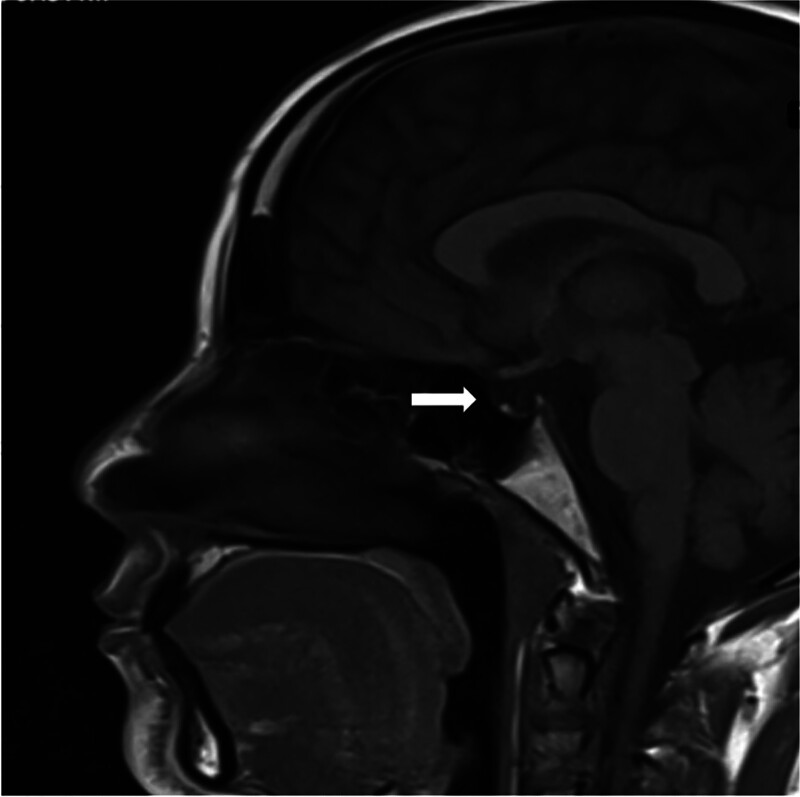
Sagittal T1W MRI image shows the sella is empty, i.e pituitary gland appears shrunken, and CSF fills the space instead (shown by white arrow).
